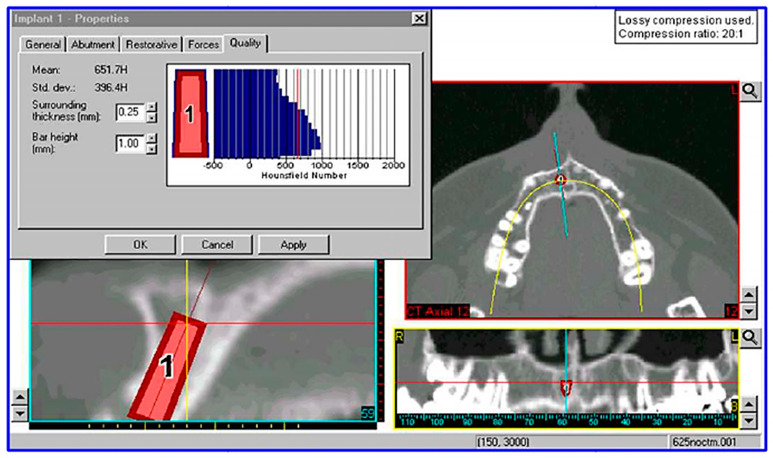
Measurement of bone density in Hounsfield Units (HU) at a virtual fixture insertion site using a computed tomography (CT) image processed with Simplant software 18.5 (Materialise HQ, Leuven, Belgium).
